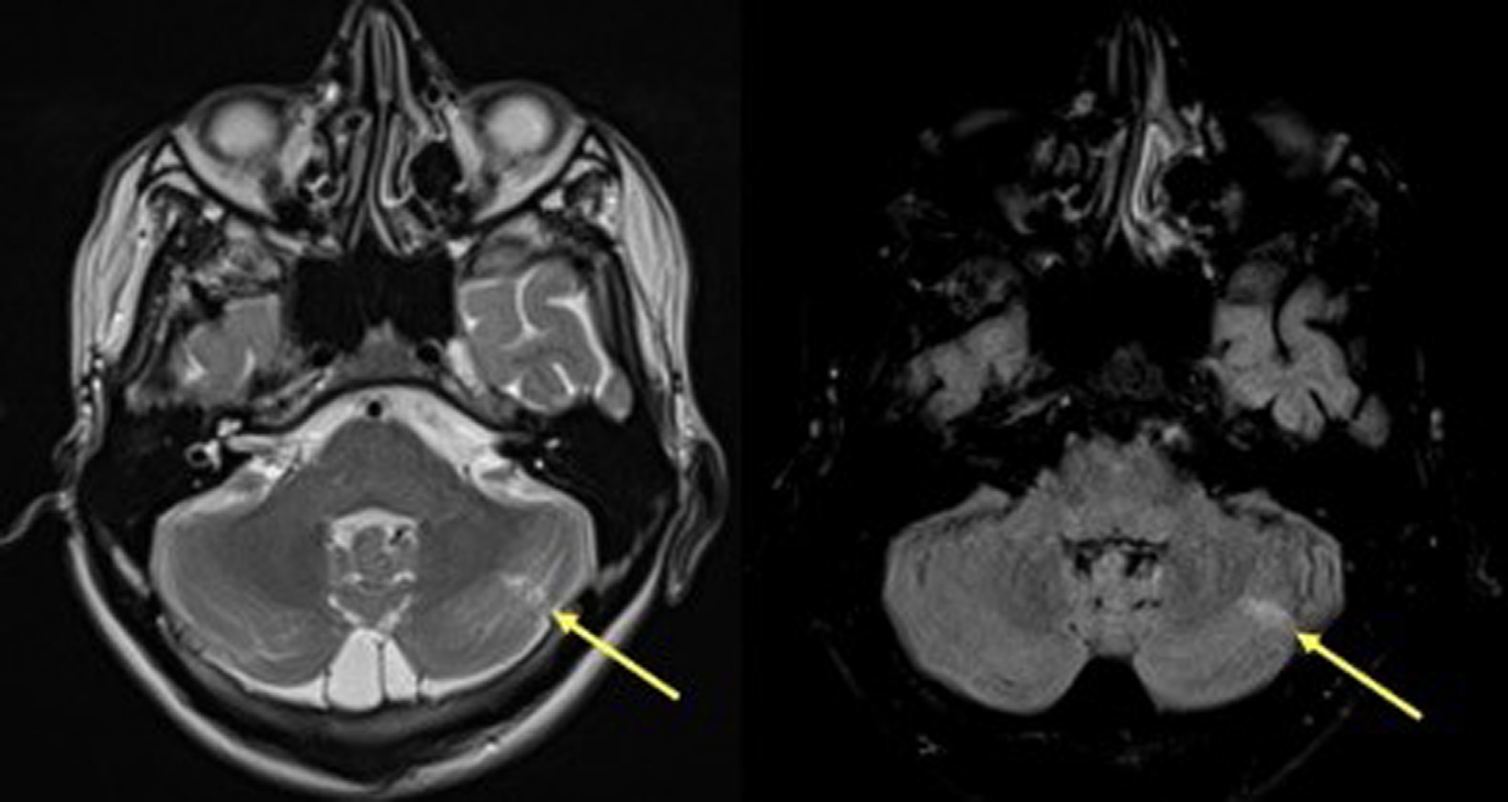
Possibility of cerebellar involvement because of diphtheria. Cerebral magnetic resonance imaging revealed 2 lacunar ischemic sequelae, one in the right posterior parietal region and the other in the left cerebellum (yellow arrows). A linear hyperintense area in T2/FLAIR, predominantly cortical, was detected in the left middle third of the cerebellum. FLAIR, fluid-attenuated inversion recovery.
